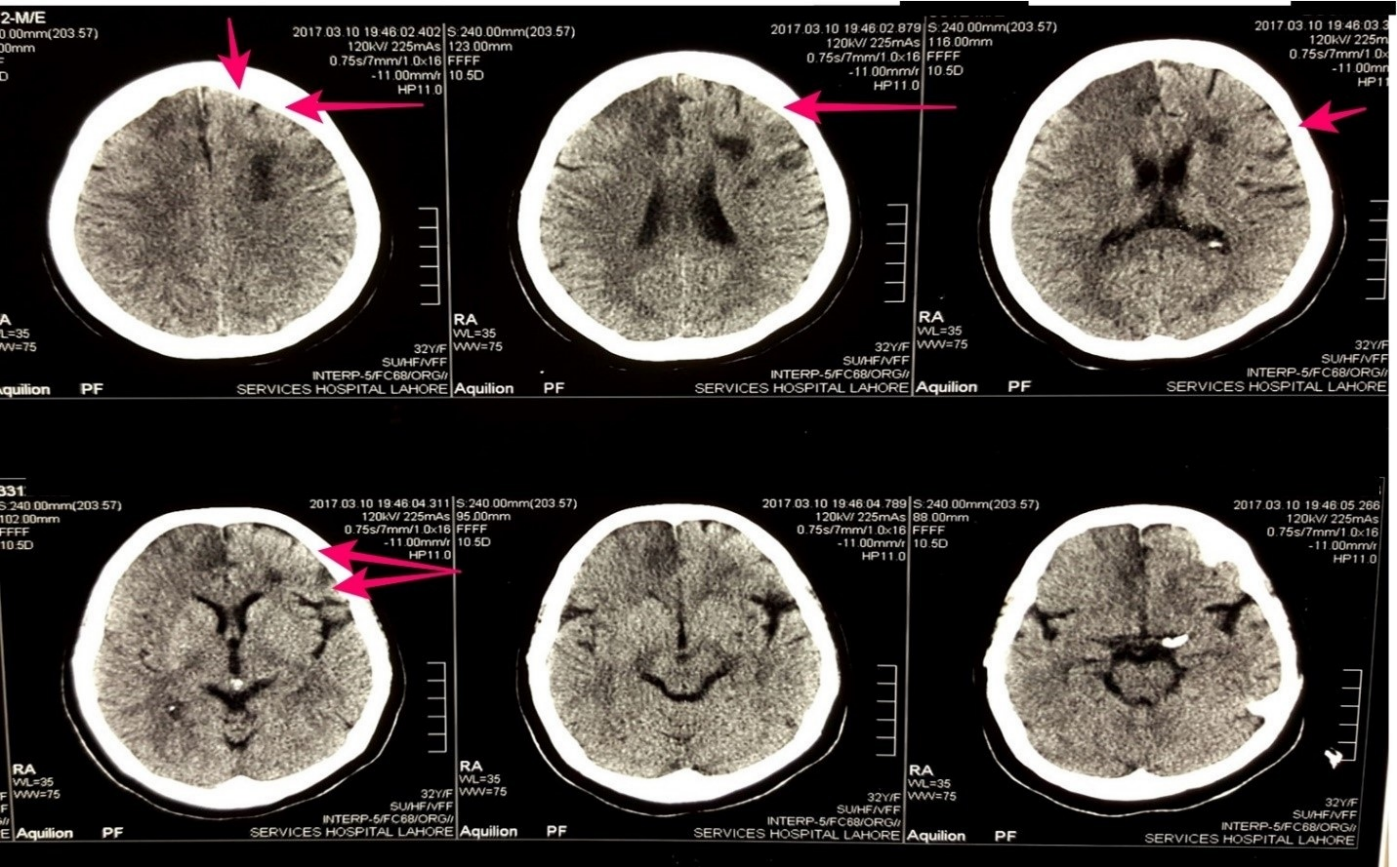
Figure showing the computed tomography scan of the patient without contrast. Red arrows show the hypodense areas in the right frontoparietal region
